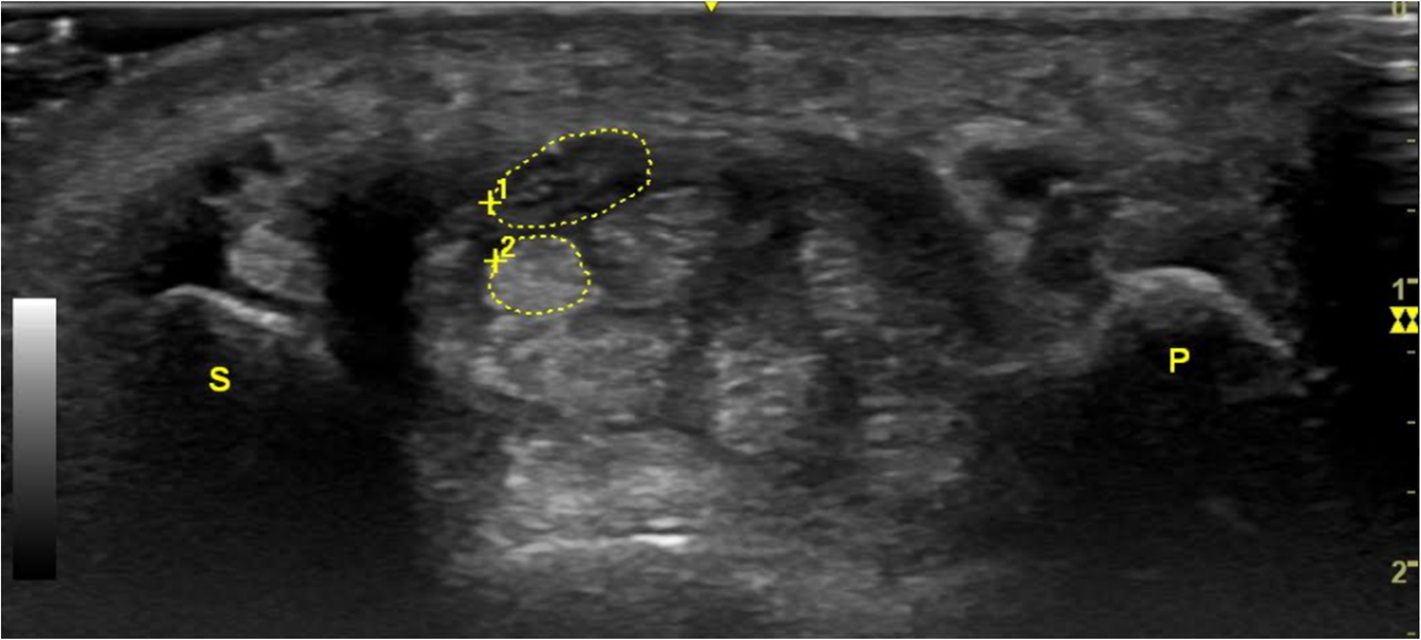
Cross sectional ultrasound image with outlined median nerve (upper encircled area) and flexor digitorum superficialis tendon of index finger (lower encircled area) at the pisiform level; P: pisiform bone; S: scaphoid bone
